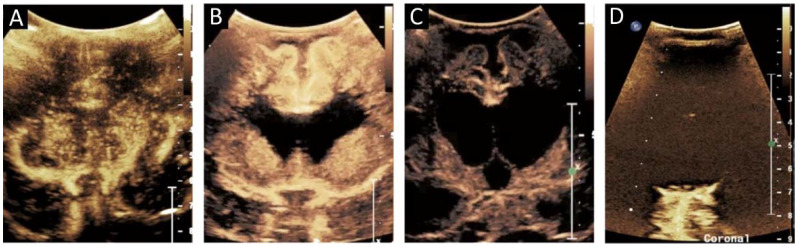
(A) Midcoronal contrast-enhanced ultrasound image of a 1 month old infant brain obtained 15 s after contrast administration reveals greater perfusion in the deep gray nuclei than in the more peripherally located white matter and the most peripheral cortex, normal for age. (B) A midcoronal brain image of a 10-month-old after cardiac arrest status post extracorporeal membrane oxygenation reveals increased perfusion in the cortex and white matter, which is atypical for an infant. The centrally located anechoic structures represent the paired lateral ventricles. (C) An image of the same 10-month-old 1 week later shows diffusely decreased brain perfusion, as well as enlarged paired lateral ventricles and the more medially located third ventricle. (D) Midcoronal image of a 6-month-old 3 h after cardiac arrest demonstrates minimal brain perfusion. Reproduced with permission from the journal Pediatrics, Vol. 143, Copyright © 2019 by the AAP.
